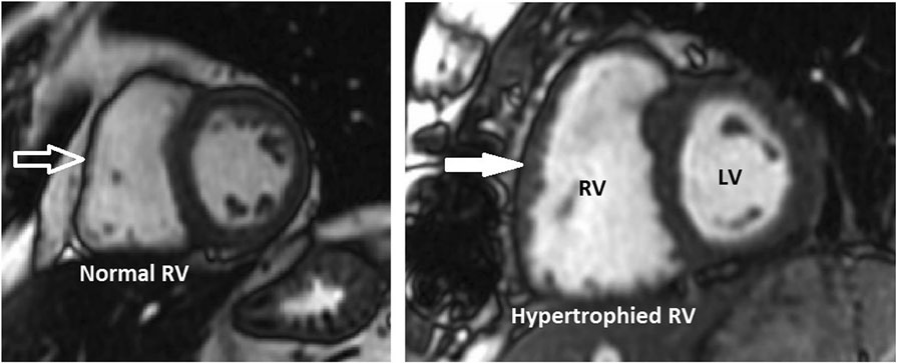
Right ventricular wall hypertrophy. Short axis SSFP MRI images demonstrate normal (open white arrow) and hypertrophied (closed white arrow) RV wall
